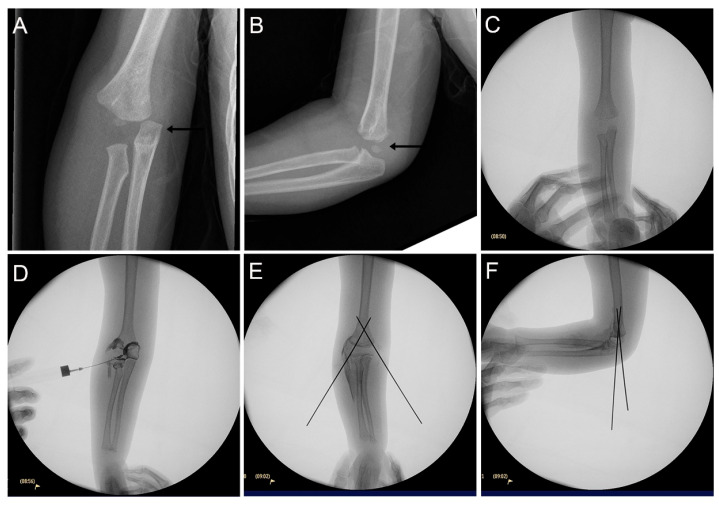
Transphyseal distal humerus fracture (TFDH) misdiagnosed as elbow dislocation. A 29-month-old girl (Case 18) was initially misdiagnosed with an elbow dislocation. (A,B): Initial anteroposterior and lateral radiographs show posteromedial displacement of the forearm relative to the distal humerus (black arrows); Note the capitellum is not yet ossified. (C–F): Closed reduction and percutaneous pin fixation performed under arthrography guidance.
